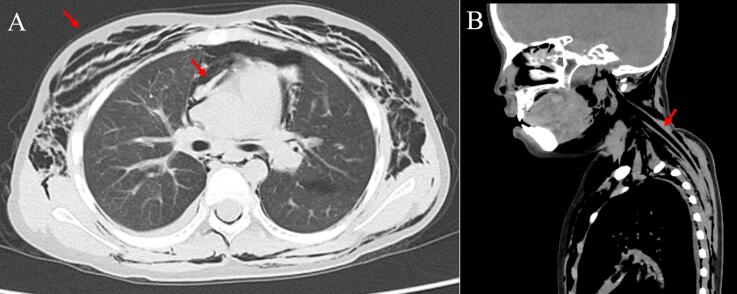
A. Axial chest computed tomography showing subcutaneous emphysema (red arrow) and pneumomediastinum (black arrow). B. Computed tomography (CT) showing a continuous distribution of air from the mediastinum to the cervical soft tissue.
